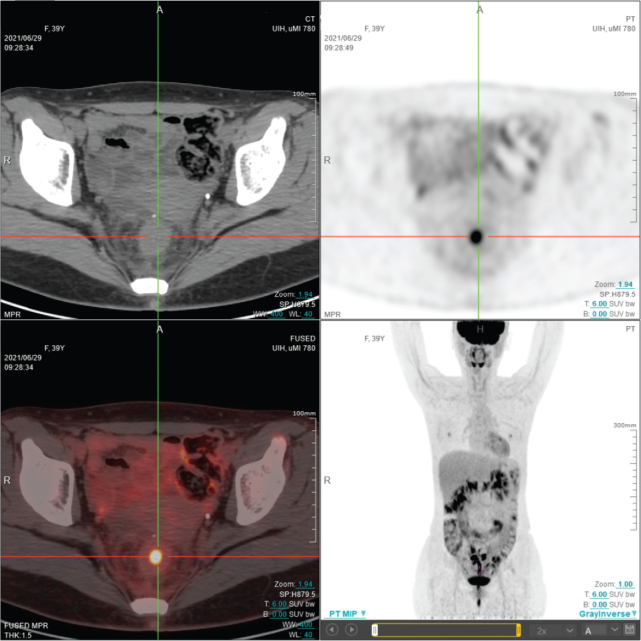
FDG-PET showed hypermetabolism in the middle section of rectum on Jun. 2021. FDG: F-18-fluoro-deoxy-glucose; PET: positron emission tomography.
